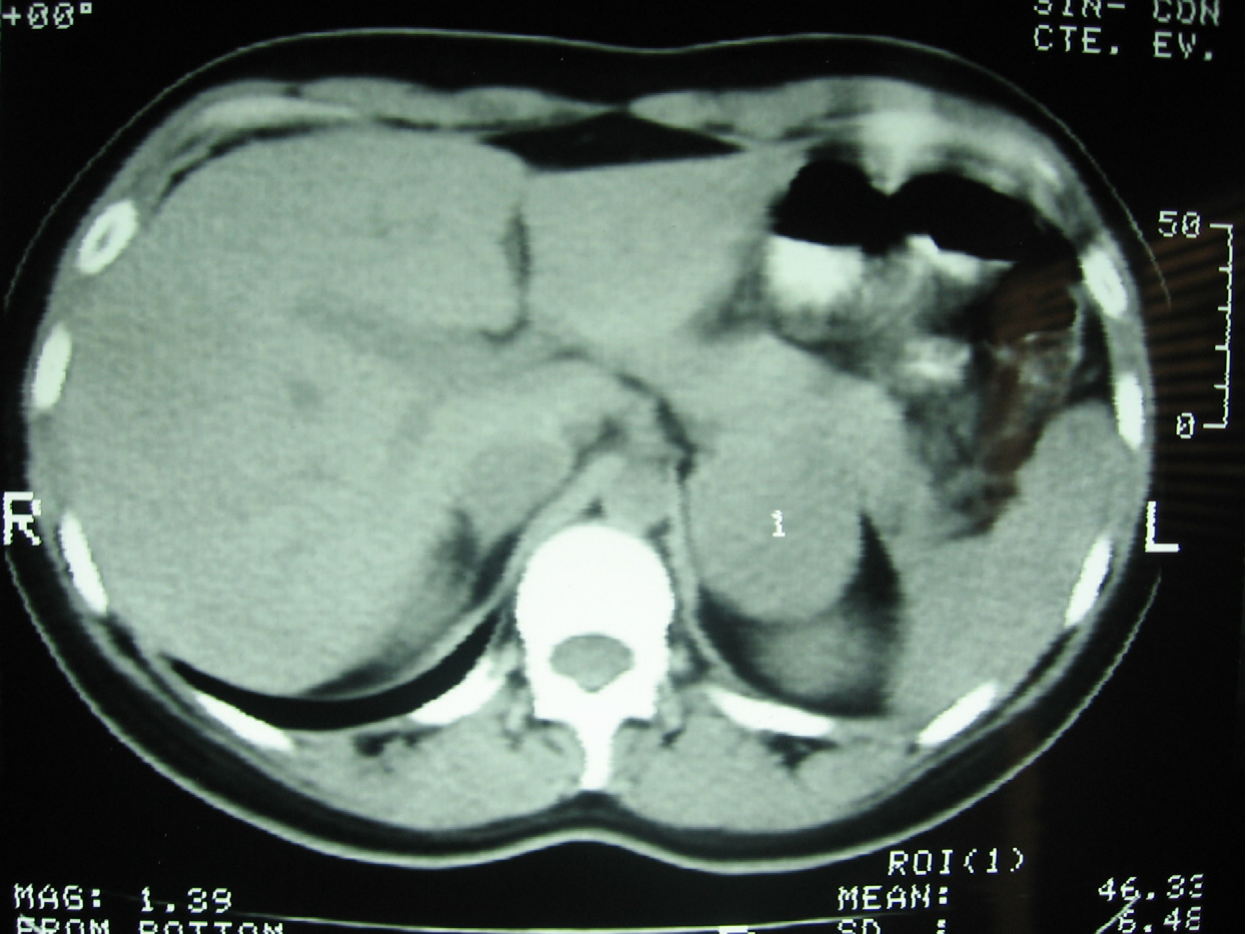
CT scan showed a 50 × 42‐mm rounded, solid, homogeneous expansive lesion of distinct borders in the left adrenal gland.
